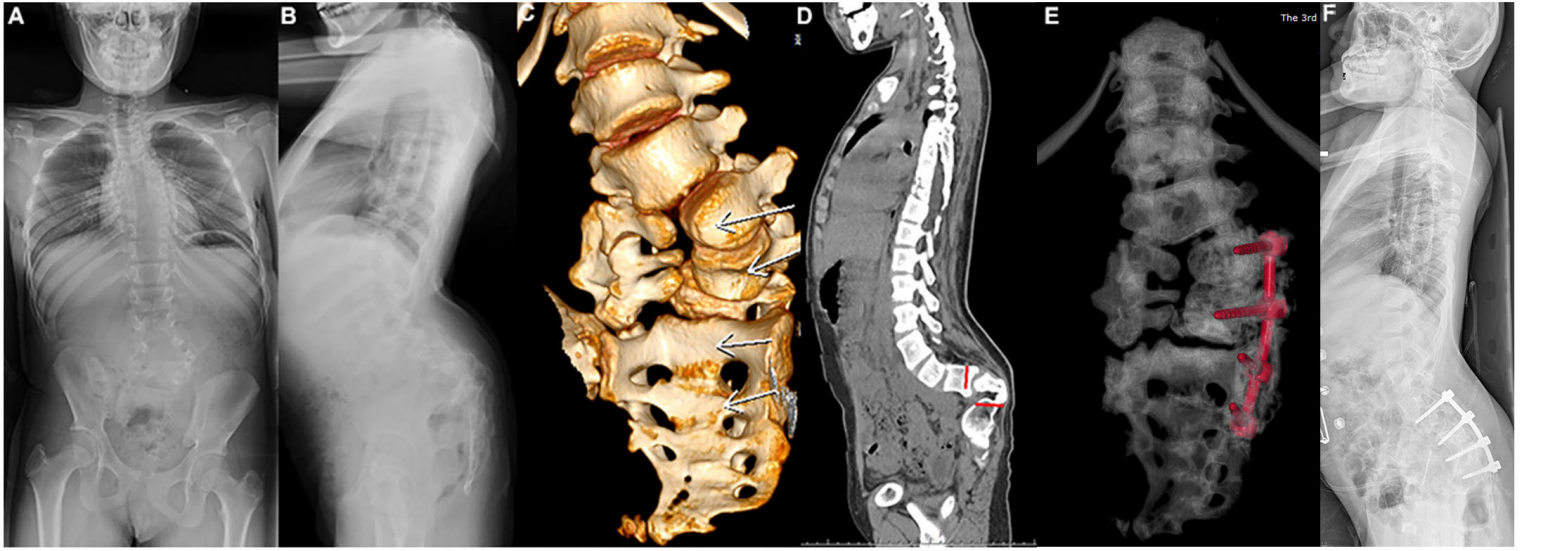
Female aged 11 years, L5–S1 hemivertebra, lumbosacral kyphosis, due to the poor development of the pedicle of the right vertebral body of L4-6, the Schwab grade 6 osteotomy of the left hemivertebral body between L5S1 and the internal fixation of the left L4, L5, S1 and S2 screw rods were performed. Preoperative x-ray [(A) coronal position, (B) sagittal position] and computed tomography (CT) (C,D), CT and X-rays after surgery (E,F).
